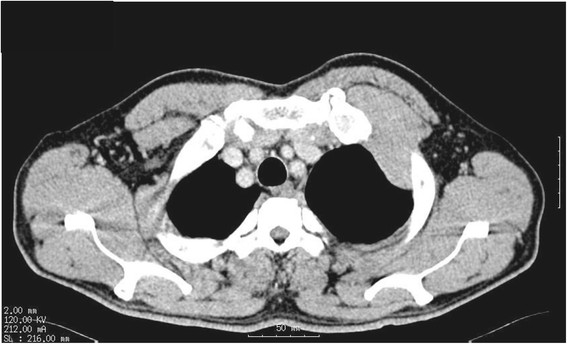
Preoperative chest computed tomography findings after the drainage of effusion. A chest wall tumor invading the space between the first and second ribs.
